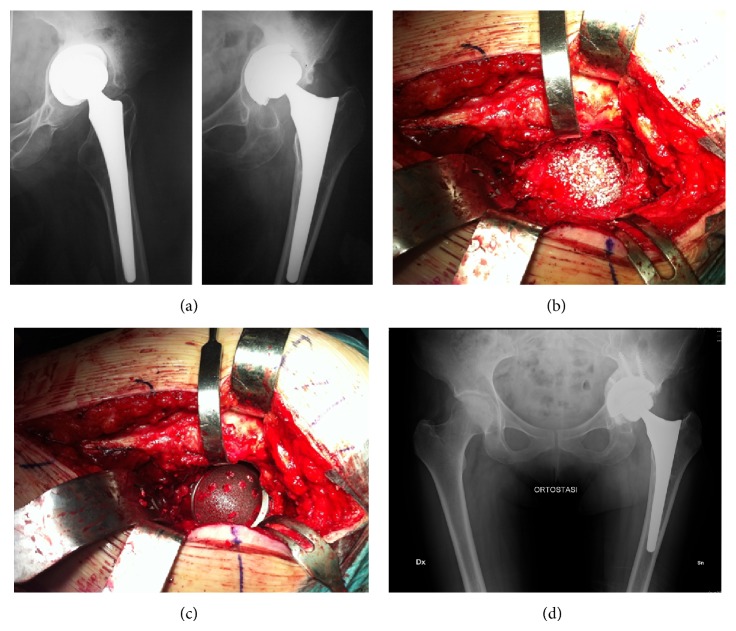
(a) Loosening of an acetabular press fit cup, stable stem. (b) Intraoperative picture showed bone defect grafted with tricalcium phosphate hydroxyapatite and morcelized bone graft. (c) Regenerex revision shell in place. (d) X-ray at the last available follow-up showing acetabular integration.
